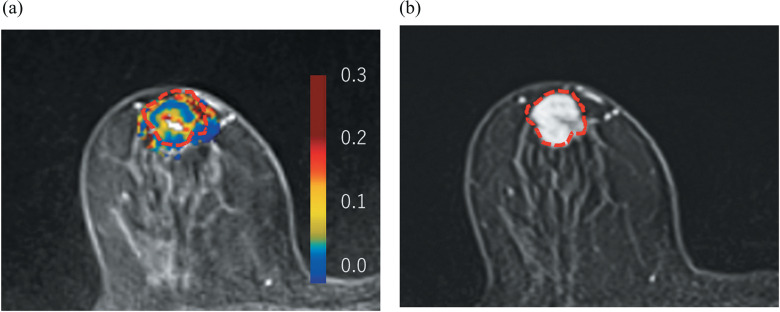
A representative Ktrans map of a woman in her 60s who underwent neoadjuvant chemotherapy for her invasive breast carcinoma in her right breast. (a) The Ktrans color map overlayed on ultrafast dynamic contrast enhanced MRI demonstrated intratumoral heterogeneous perfusion, with a mixture of high Ktrans (yellow & red) and low Ktrans (blue) within the lesion (indicated by the red-dotted line). Median of Ktrans was 0.18/min. (b) Initial phase of dynamic contrast enhanced MRI of the same lesion demonstrated more homogeneous enhancement pattern.
